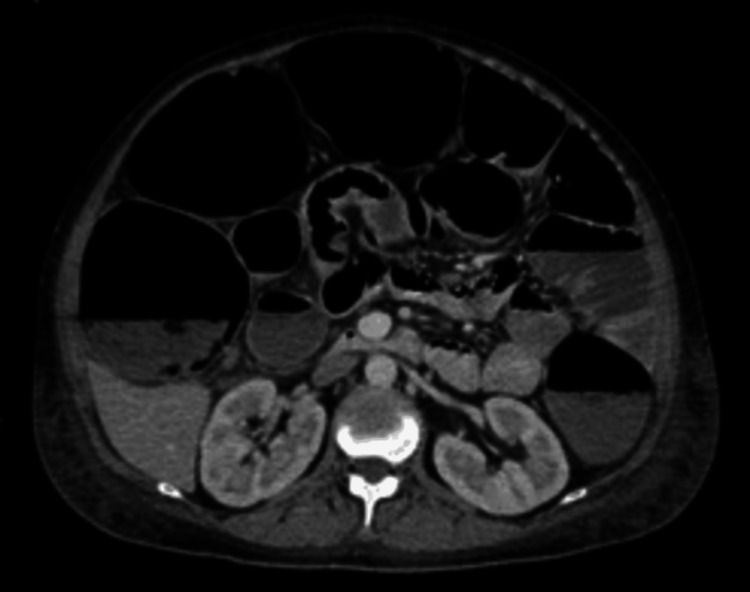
Contrast-enhanced computed tomography (CECT) of the abdomen showed diffuse small bowel dilatation measuring up to 4.3 cm and large bowel dilatation measuring up to 7.5 cm, with no identifiable transitional point.
